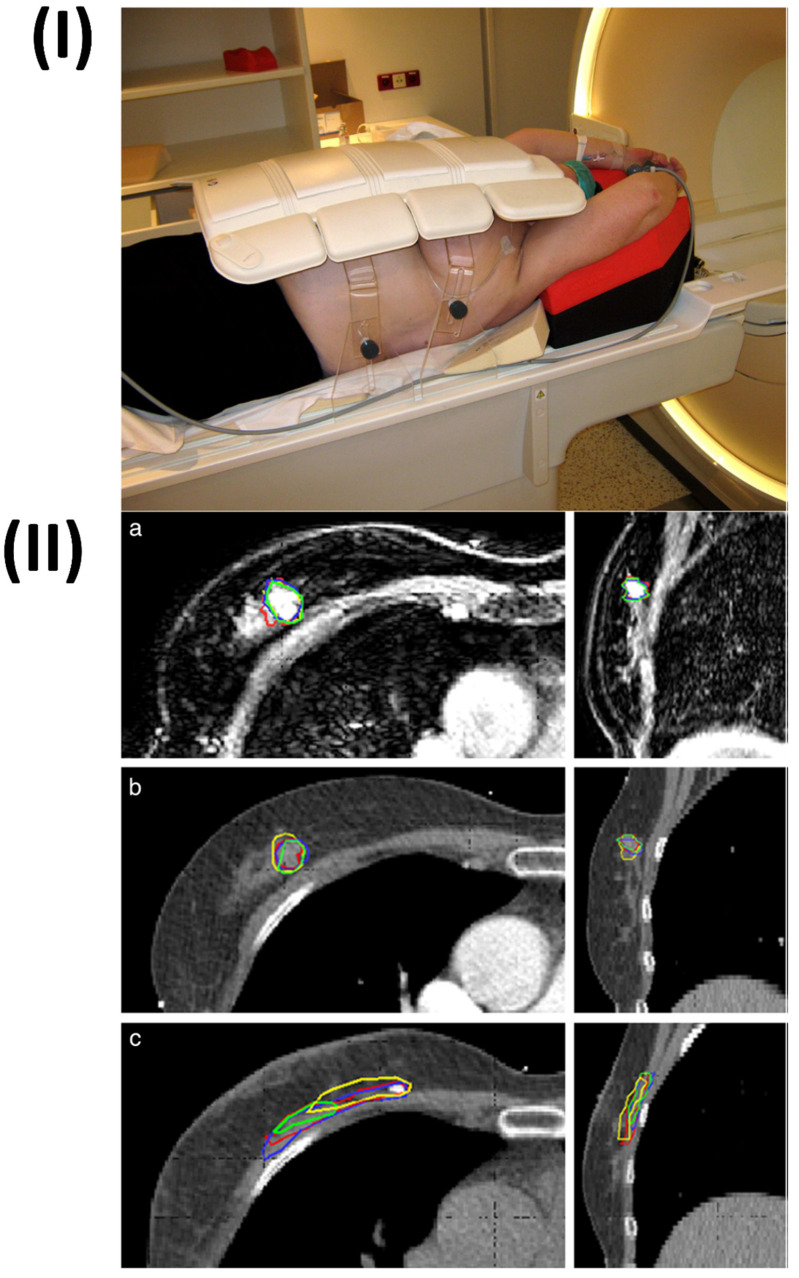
(I) MRI patient positioned in the supine position for radiation therapy; (II) 3D GTV outlines in the sagittal and transversal planes as seen by four separate observers on a single patient (a) Preoperative CE-MRI (b) Preoperative CE-CT (c) Clinical postoperative CT. Reproduced with the permission form ref. 161 Fig. 1, Fig. 3 (Springer, 2014©).
